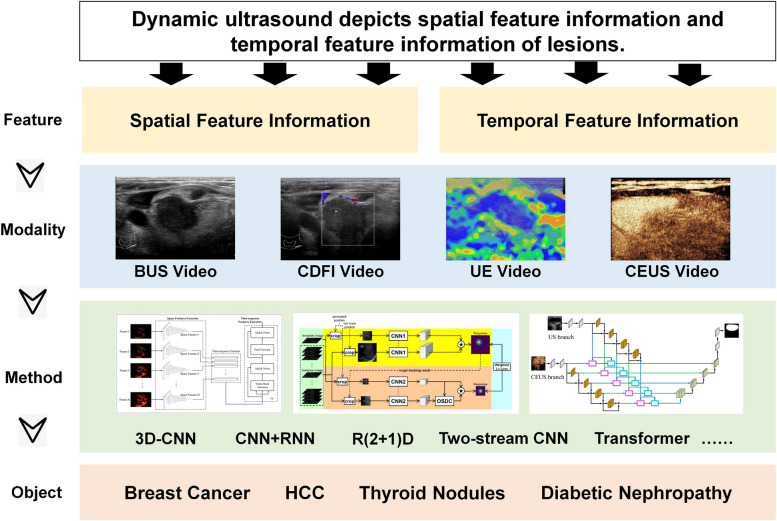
Dynamic ultrasound analysis model for diagnosis and prediction. Dynamic ultrasound reflects the spatiotemporal characteristics of lesions. It involves videos. Common ultrasound modalities include BUS, CDFI, UE, and CEUS. Current studies on ultrasound videos primarily focus on BUS and CEUS videos. The commonly used intelligent analysis methods for ultrasound videos include three-dimensional CNN, CNN + RNN, R(2 + 1)D, dual-stream CNN, and transformers. These are frequently employed for the diagnosis and prognosis of diseases such as breast cancer, liver cancer, thyroid nodules, kidney disease, and diabetes
